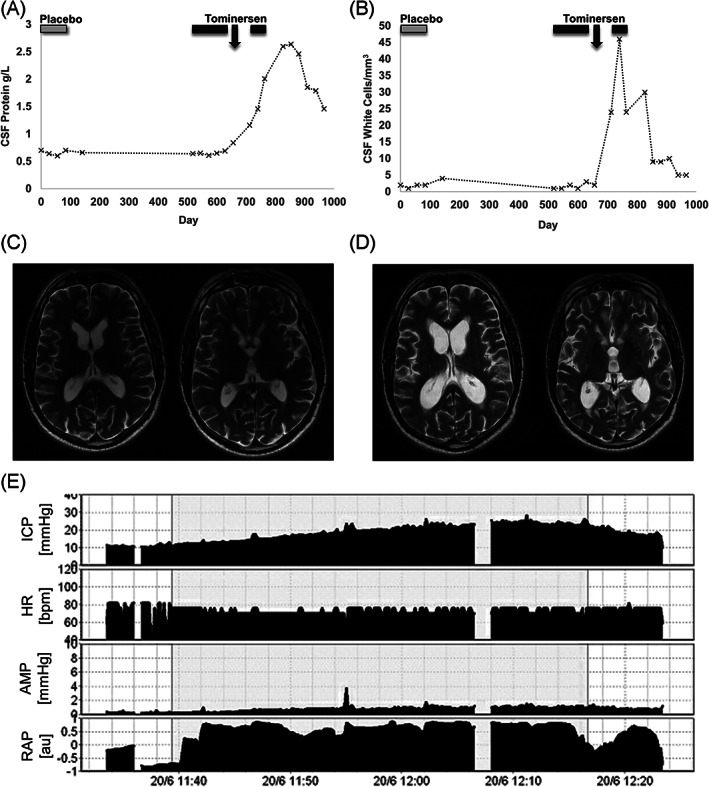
Investigation results. Cerebrospinal fluid (CSF) protein (A) and leukocyte count (B). Light and dark gray bars represent treatment with placebo and tominersen, respectively. Arrow indicates clinical deterioration. Axial T2‐weighted magnetic resonance imaging at baseline (C) and after deterioration (D) Lumbar infusion study (E) AMP, pulse amplitude; HR, heart rate; ICP, intracranial pressure; RAP, compensatory reserve index.
